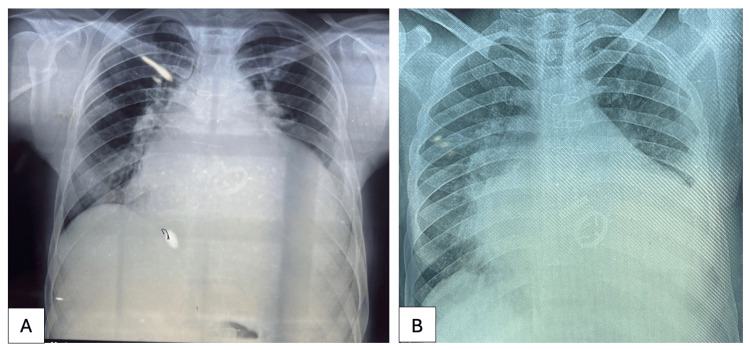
Chest radiographs: posteroanterior view A. Two years after the cardiac surgery (depicting Gross cardiomegaly, sternal sutures and prosthetic cardiac valves in situ) B. Four years and five months after the cardiac surgery (depicting Gross cardiomegaly, sternal sutures and prosthetic cardiac valves in situ, along with ground-glass opacities in bilateral lung fields)
